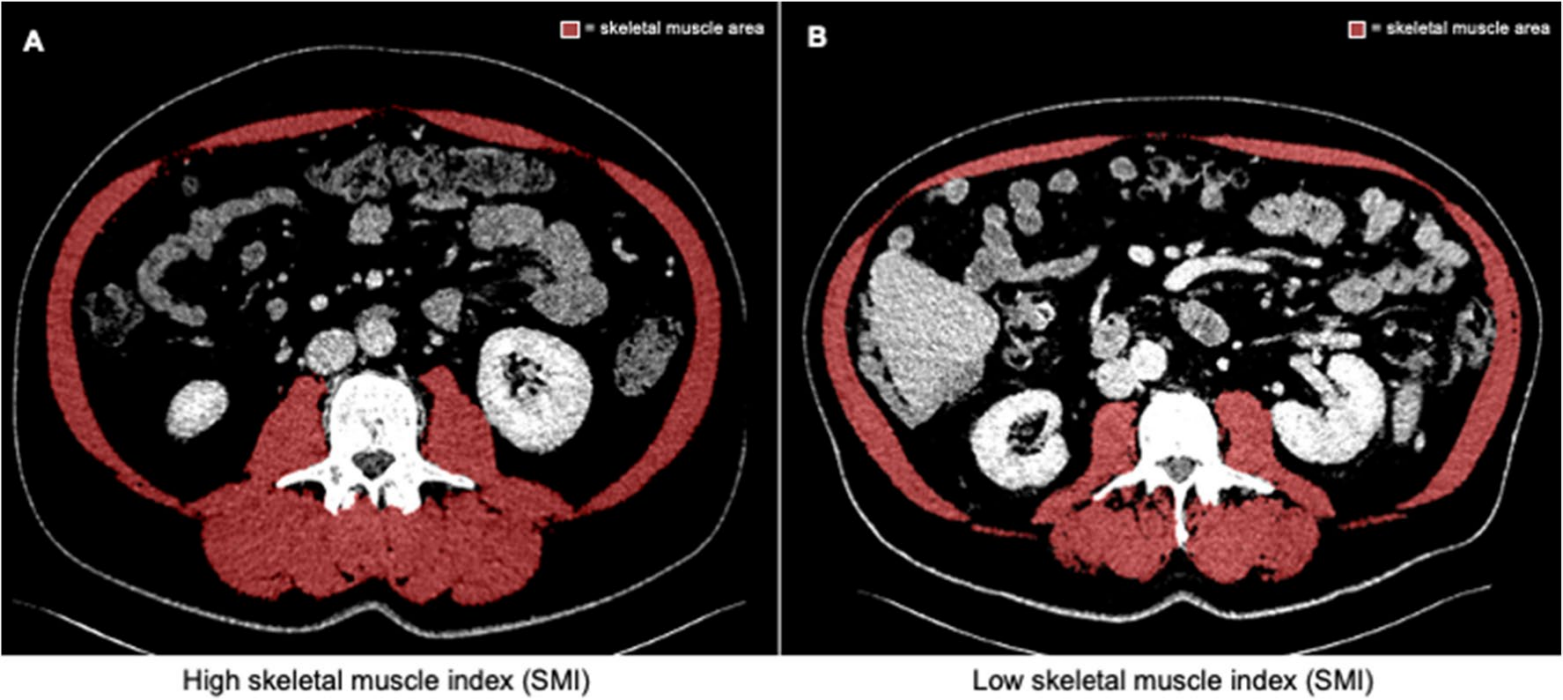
Measurement of the SMI using routine CT scans. (A) Example presentation of a patient with a high SMI. B Example presentation of a patient with a low SMI
